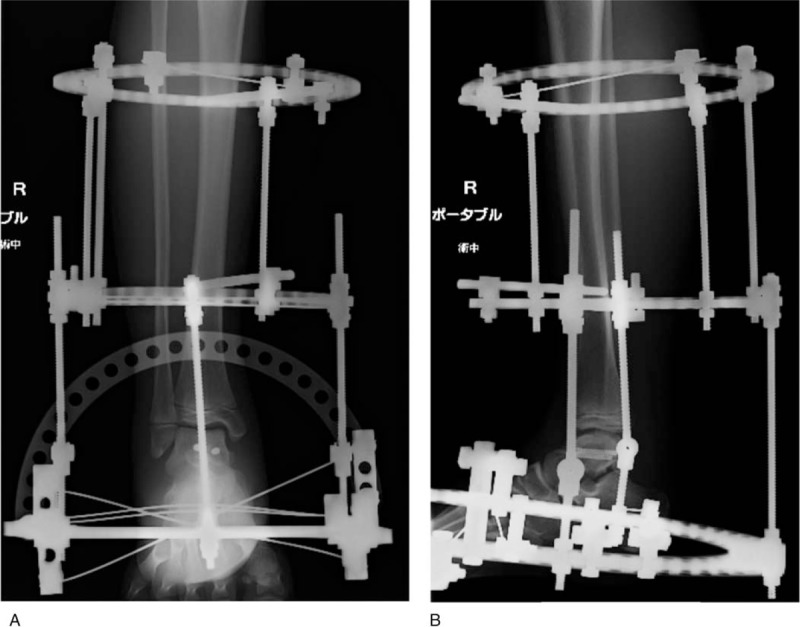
Postoperative radiographs. (A) Anterior-posterior view. (B) Lateral view.
